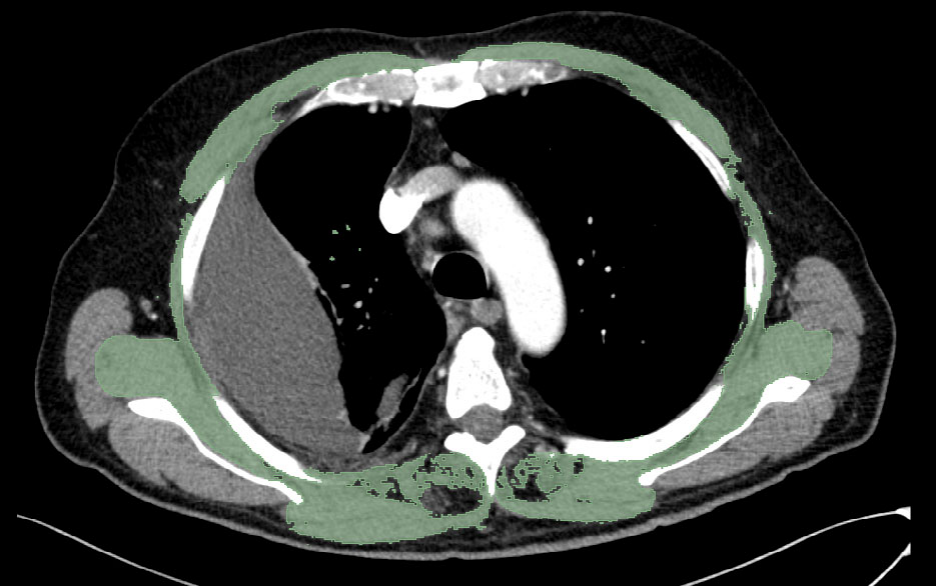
Sample axial chest CT image of the fourth thoracic vertebral region. Colored (green): cross sectional area of pectoralis, intercostalis, paraspinal, serratus, and latissimus muscles determined by semiautomatic muscle mass quantification. CT, computed tomography.
